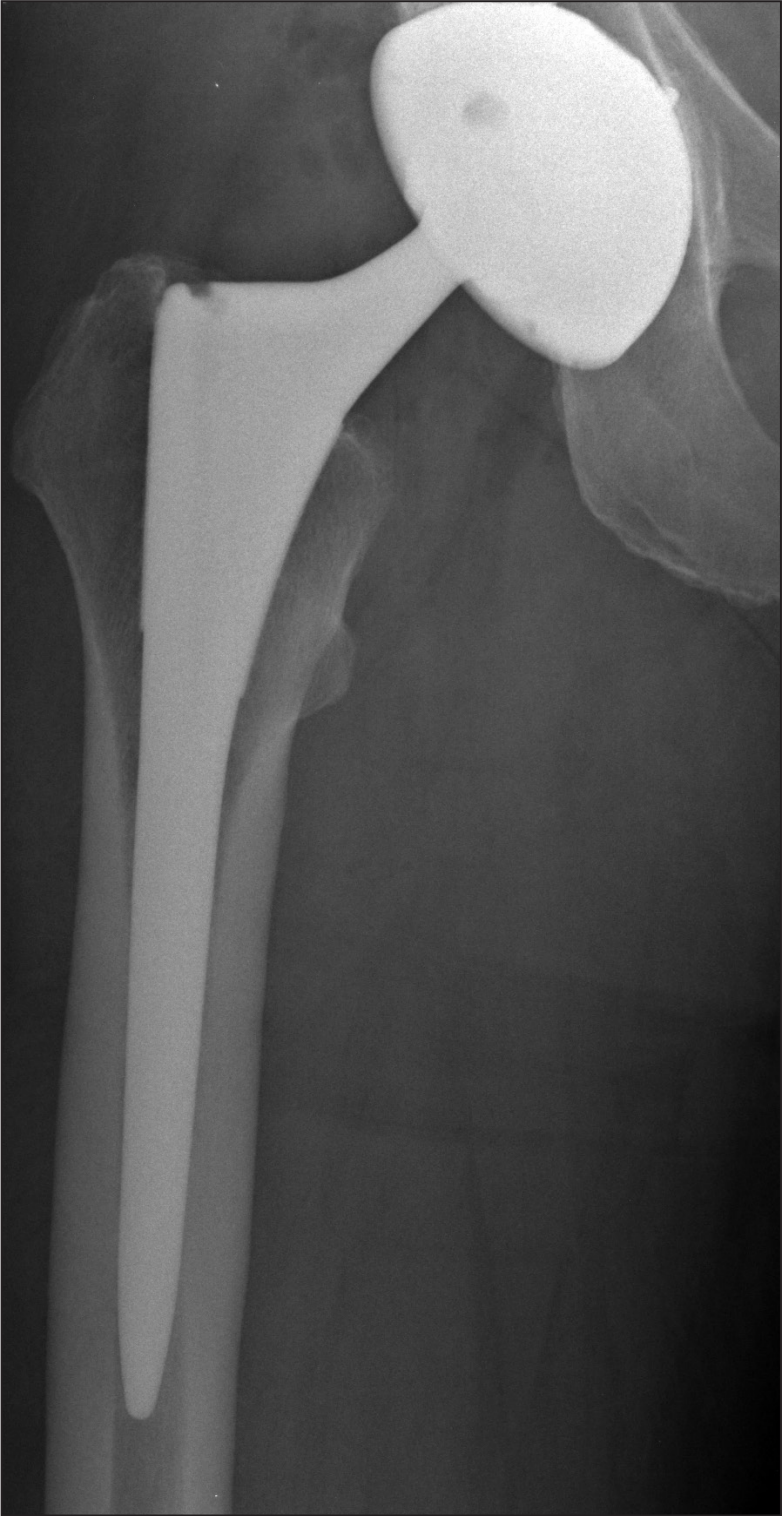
Radiograph of a well-fixed, tapered, cementless femoral stem at an eight-year follow-up
